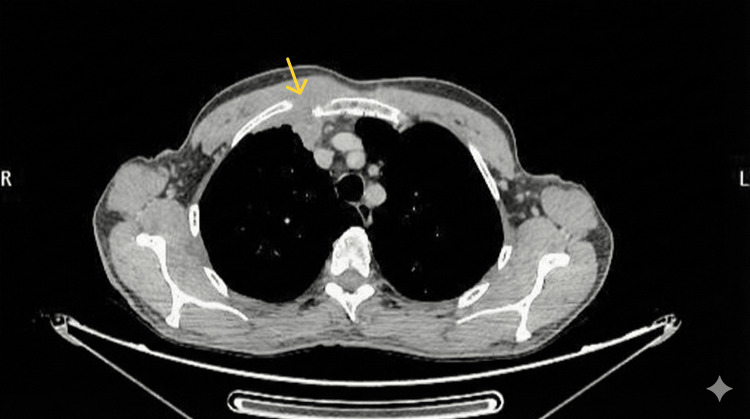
Axial CT scan image showing the sternal mass of Case 1. The yellow arrow depicts the sternal mass.
